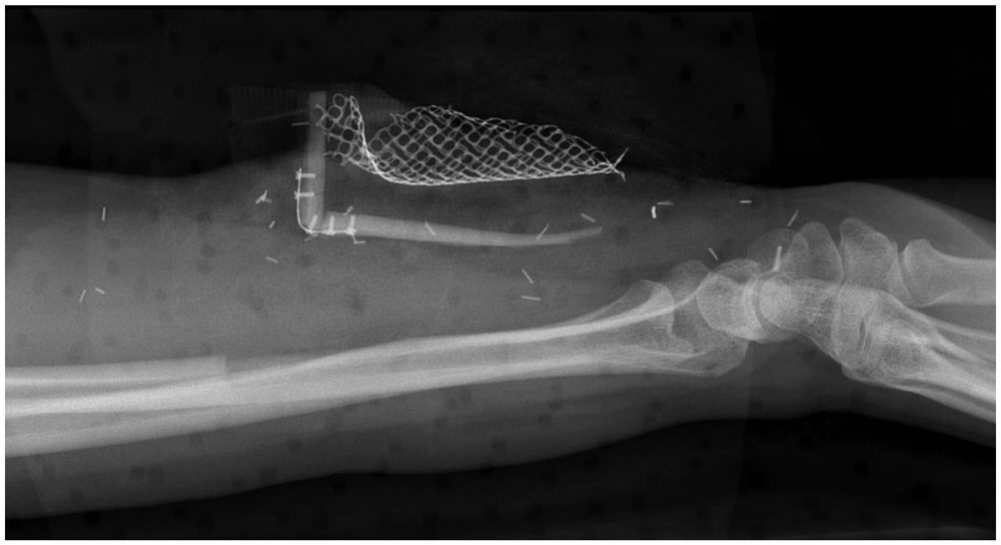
X-ray of the patient forearm after the 1st stage. Note the “L-shaped” bone framework and the position of titanium coated cage.
